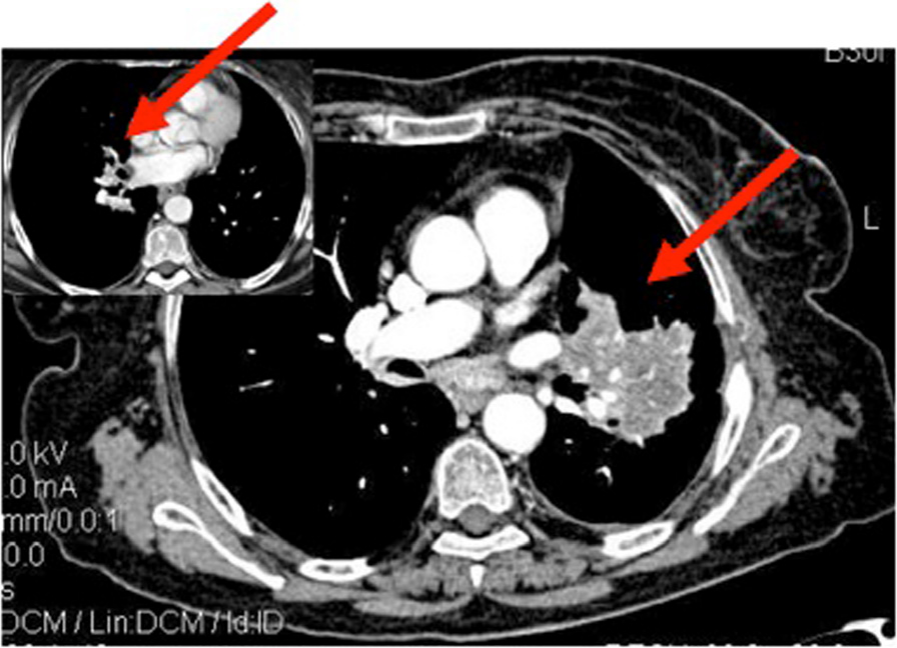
Thorax-abdomen-pelvis computed tomography two months after diagnosis, showing progressive disease.
